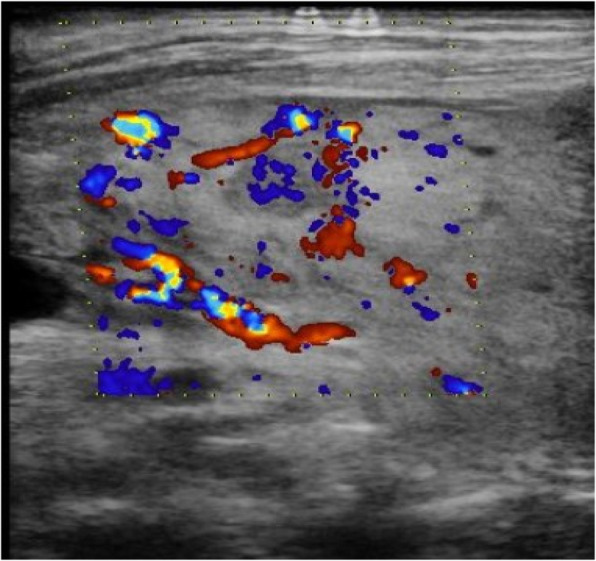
Color Doppler ultrasound of thyroid gland of the patient
